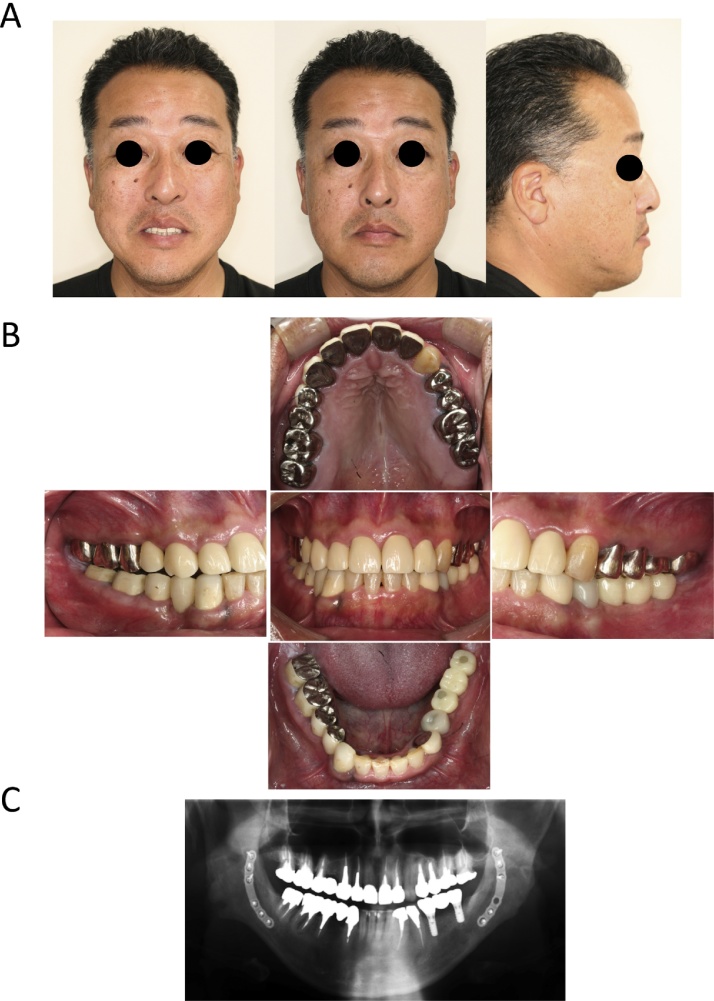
Extraoral and intraoral photographs and panoramic X-ray at debonding in Case 1.
